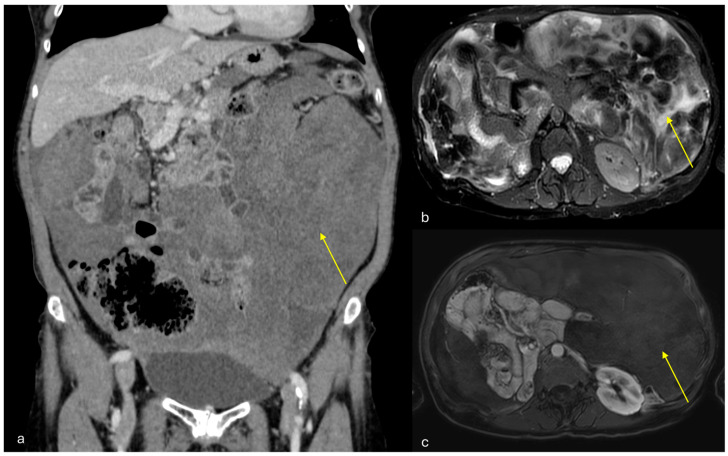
Angiomyofibroblastoma. A middle-aged woman. CT and MRI show a voluminous non-infiltrative peritoneal and pelvic lesion encasing the small bowel loops ((a): coronal portal venous phase CT; (b): axial T2-weighted MRI; (c): axial venous phase MRI. Indicated by arrows).
